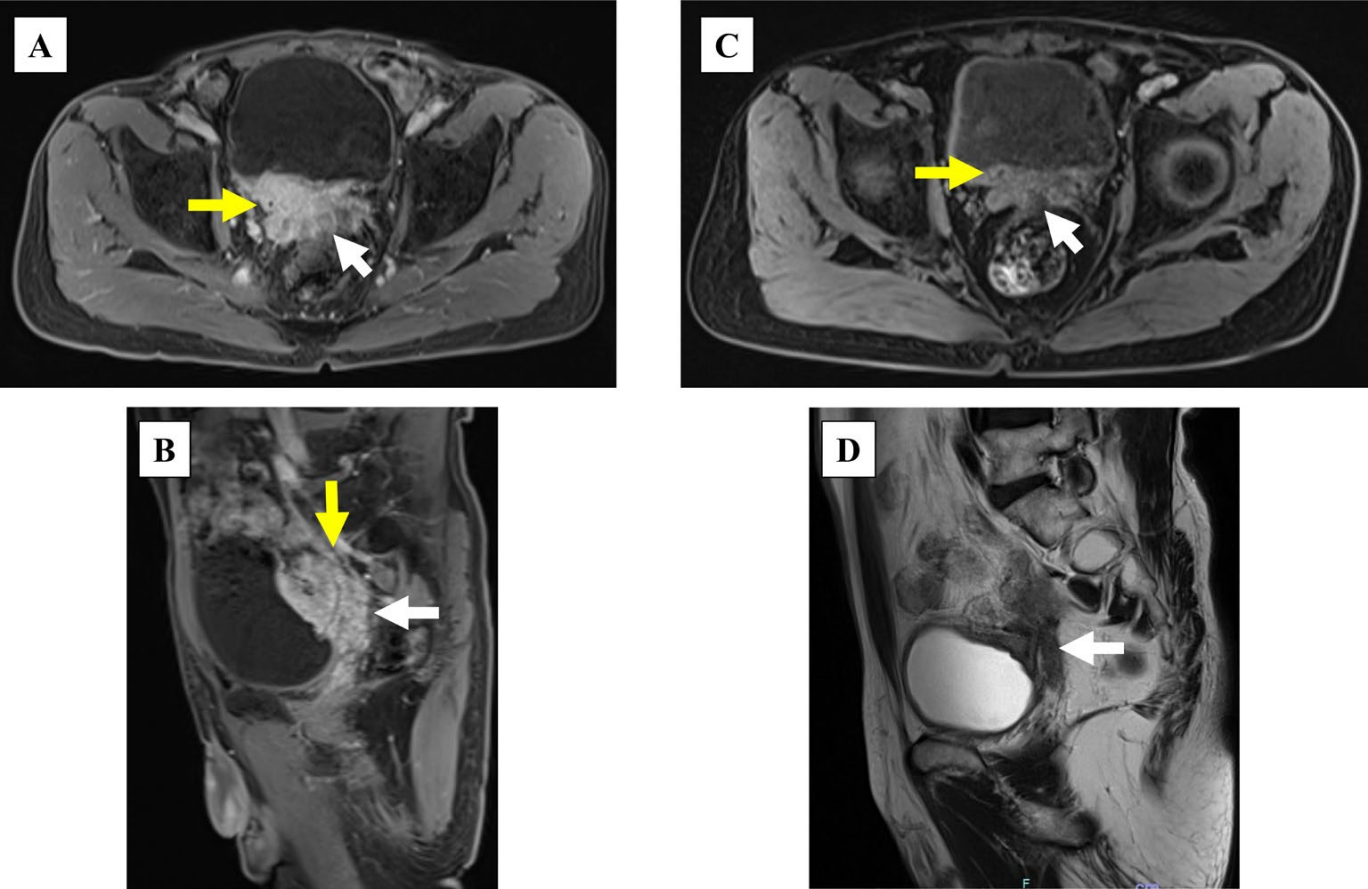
Magnetic resonance imaging of the submucosal bladder mass. Axial (A) and sagittal (B) T1‐weighted images with contrast showed a submucosal bladder mass (white arrow, 65 × 40 × 40 mm^3^). Axial T1‐weighted (C) and sagittal T2‐weighted (D) images showed shrinkage of the submucosal bladder mass (white arrow, 10 × 10 × 10 mm^3^) after two cycles of neoadjuvant chemotherapy. The yellow arrows indicate the right ureter.
